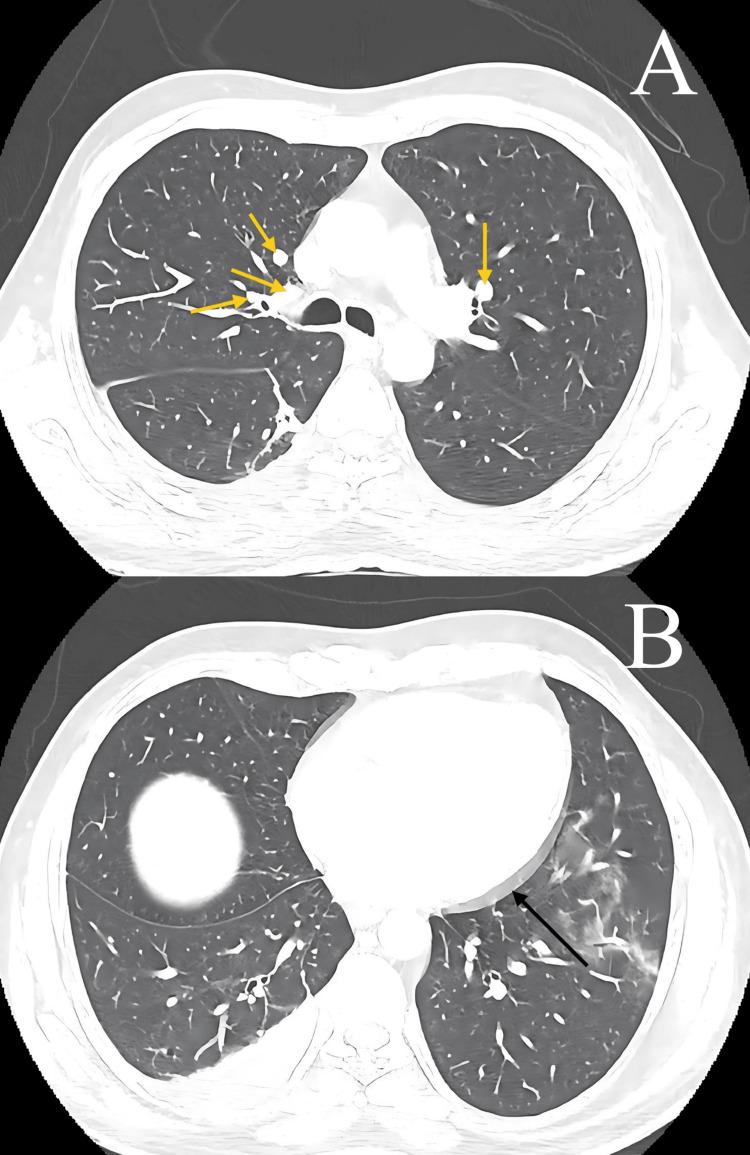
Chest computed tomography (A) Mediastinal lymphadenopathy (yellow arrows). (B) Pericardial effusion (black arrow).
